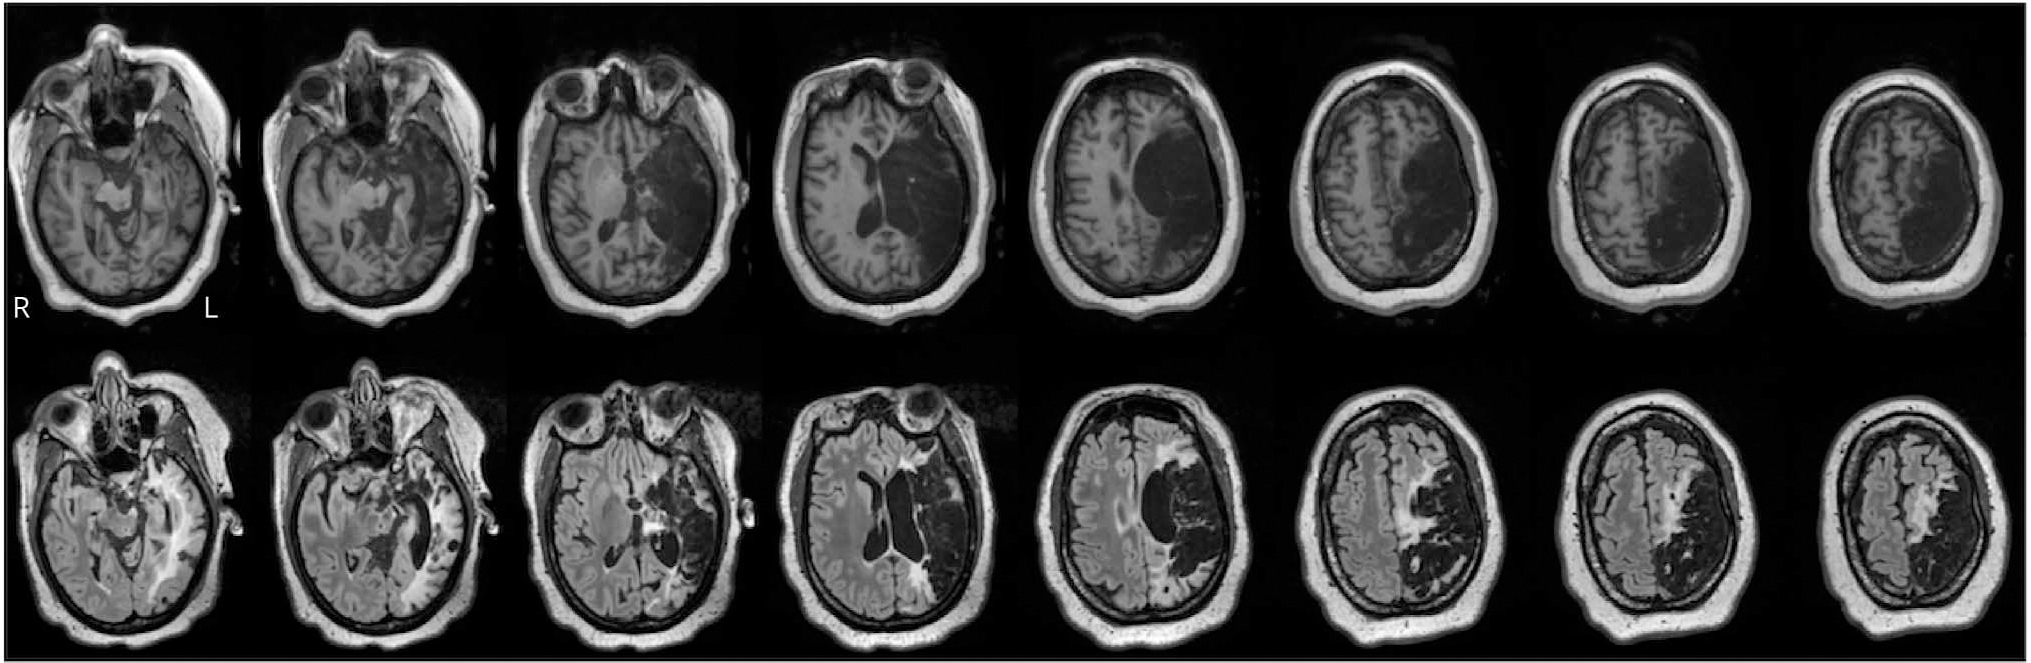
MRI Acquired 61-Month Poststroke Flair and T1-weighted MRI acquired 61-month poststroke showing left hemisphere stroke encompassing MCA and posterior ACA territories. ACA = anterior cerebral artery; MCA = middle cerebral artery.
